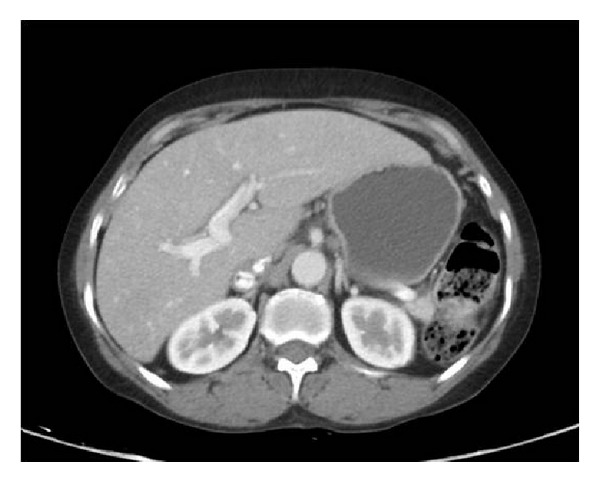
CT of abdomen showing hepatomegaly.
